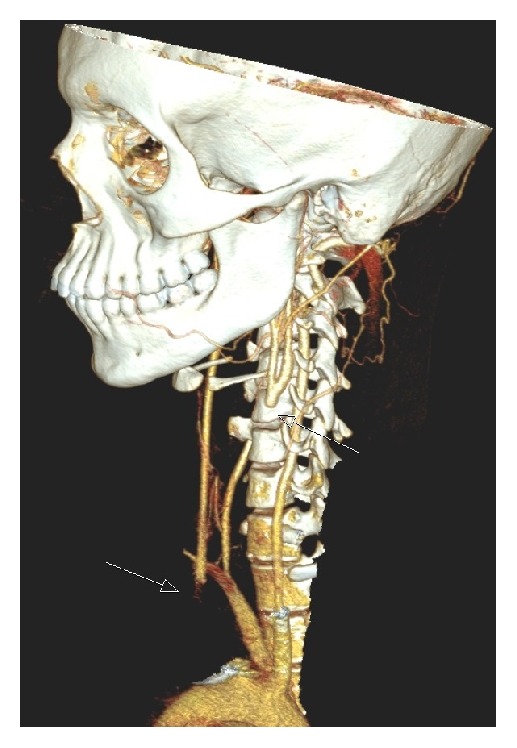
Spiral CT angiography displaying a high-grade stenosis at the origin of the right common carotid artery, whereas the left common carotid artery is occluded.
